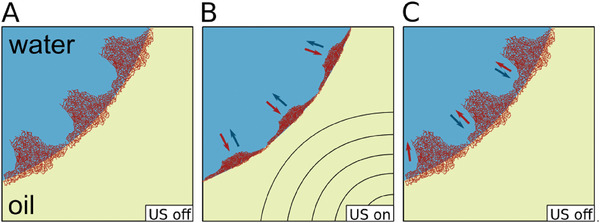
Proposed model to describe the behavior of microgels at the oil water interface under the influence of ultrasound. A) Ultrasound off: microgels adsorb spontaneously at the interface of the drop and reduce the interfacial tension until the drop is fully covered. B) Ultrasound on: Under the influence of ultrasound the microgels are shrinking mostly horizontally to the interface and the water is pushed away from the interface. The interfacial tension decreases. C) After ultrasound is turned off the microgels relax in their initial state similar to (A). The interfacial tension also relaxes to the initial value. The blue arrows indicate the direction of the water and red arrows show the direction of shrinking/swelling of microgels.
